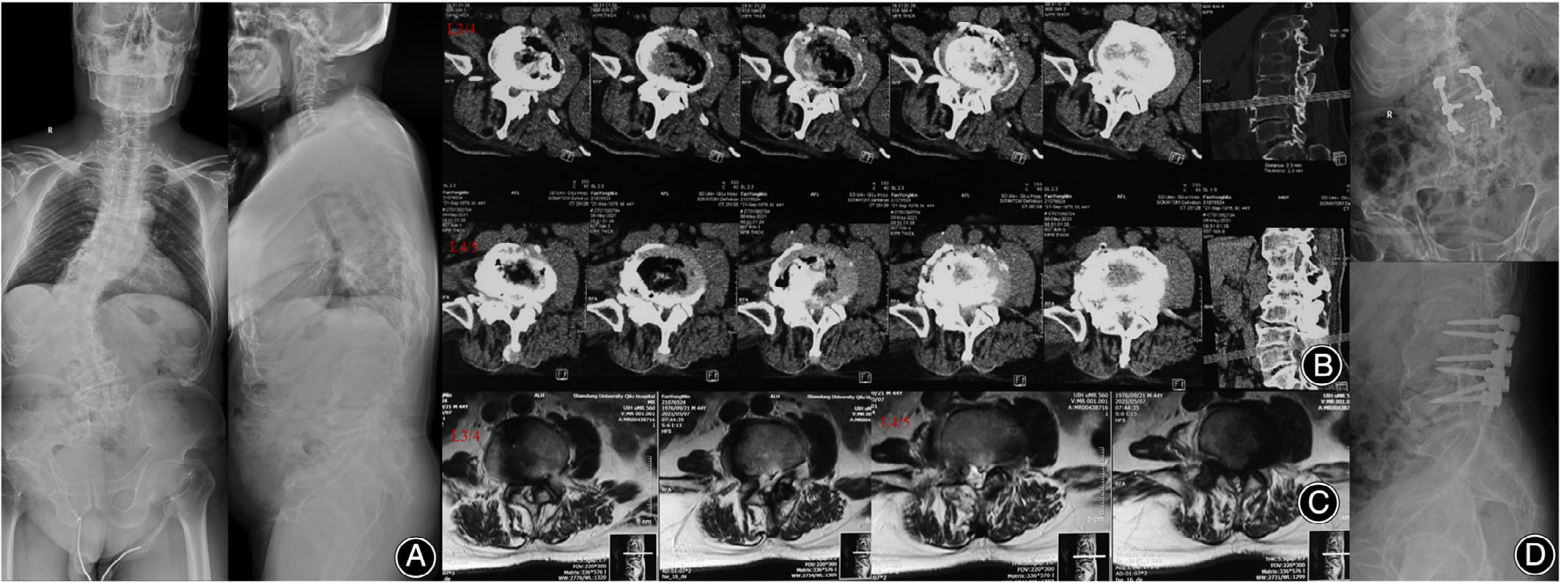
Radiographic data prior to operation in case 2. PA X‐ray image shows thoracolumbar scoliosis with a cobb angle of 65° (A). Axial CT and MR images show L4/L5 disc herniation with L3/L4 and L4/L5 segmental spinal stenosis (B, C). (D) presents the postoperative lumbar spine X‐ray image.
